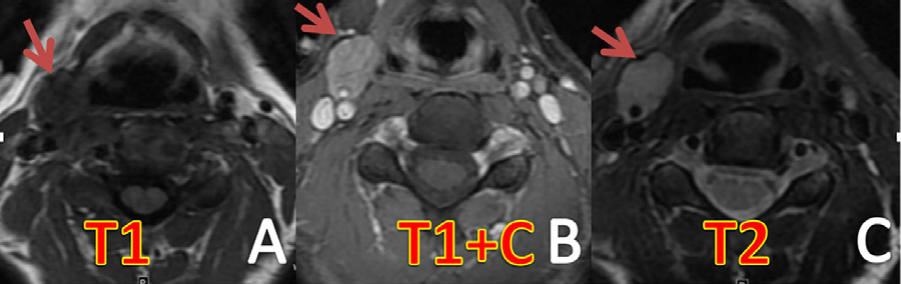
Axial T1-weighted (A), gadolinium-enhanced axial T1-weighted (B), and axial T2-weighted (C) MR images of the neck demonstrating homogenously enhancing approximately 2 cm minimally lobulated T2 hyperintense mass anterior to right common carotid artery bifurcation, contacting and likely encasing, but not significantly narrowing the proximal right external artery.
